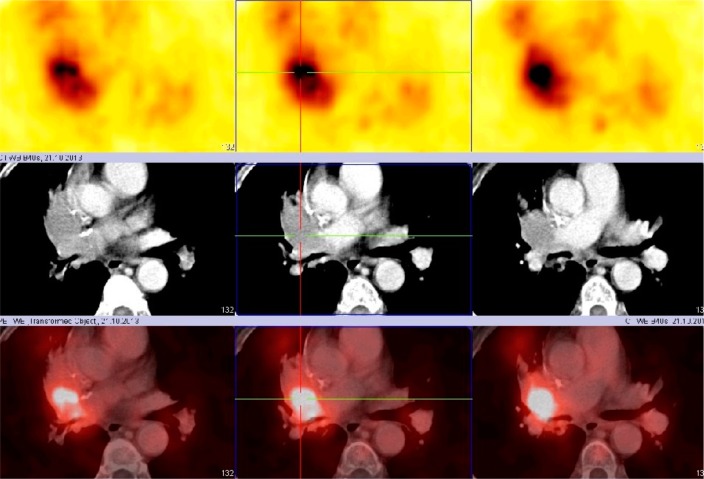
^68^Ga-CPCR4-2 PET/CT (transversal images): local recurrence of an centrally localized SCLC (upper panel: PET scan, middle panel: CT scan, lower panel: PET + CT fusion image).
